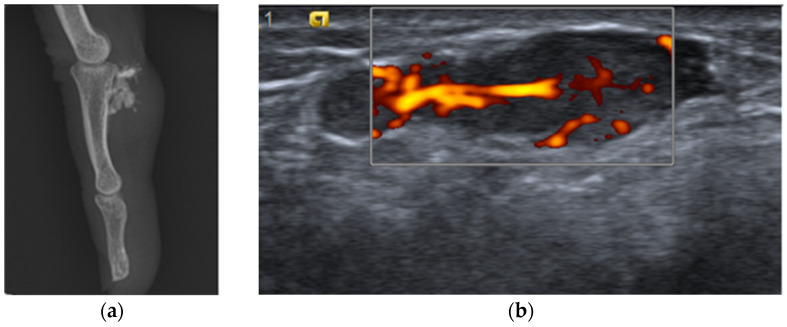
Radiological and ultrasound characteristics: (a) X-ray showing calcification of the lesion, and (b) ultrasound showing a well-circumscribed lesion hyperechogenic with hypervascularity on Doppler.
